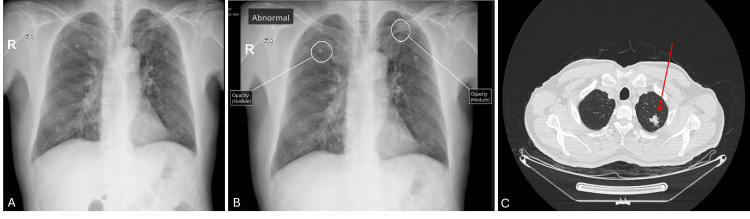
Chest X-ray and CT images of patient 2 A: Chest X-ray image without AI; B: Interpretation of chest X-ray with AI (suspicious nodules marked); C: Computed tomography (CT) image of the thorax (arrows show nodules)
